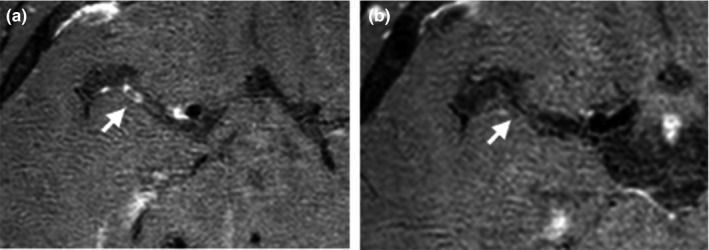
(a) Male, 46 years old, HR‐MRI showed diffuse uniform thickening and strengthening of the M1 segment of the right middle cerebral artery. (b) After treatment, the enhancement of the wall disappeared
